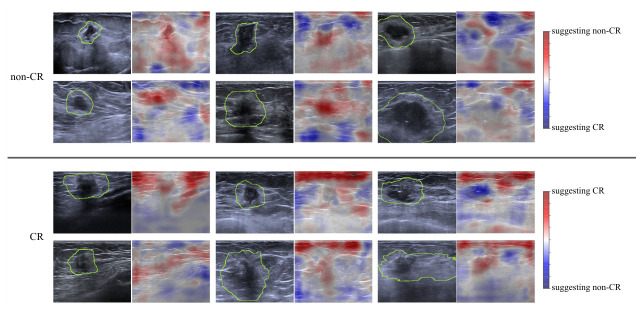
Grad-CAM visualizations for treatment response prediction from breast ultrasound images. The rows show six representative examples from patients predicted as non-complete responders (non-CR) and complete responders (CR). For each example, the original ultrasound scan is displayed, with highlighted tumor and peritumoral position, as well as with a Grad-CAM heatmap overlay, highlighting image regions that contributed most to the model’s prediction. The heatmap indicates that, for non-CR cases, the model focused on the tumor and the retrotumoral posterior shadowing, whereas, for CR cases, it emphasized more superficial peritumoral regions with less attention to the tumor itself.
